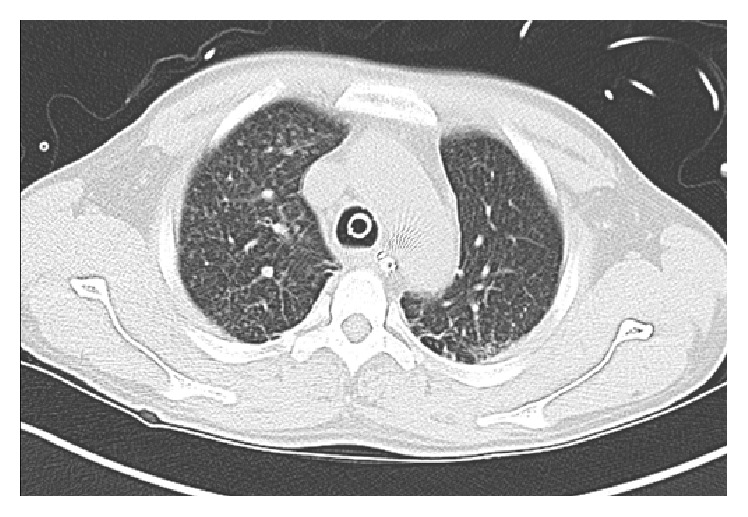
Chest CT scan showing numerous randomly distributed nodules bilaterally in upper lobe consistent with miliary pattern of distribution.
